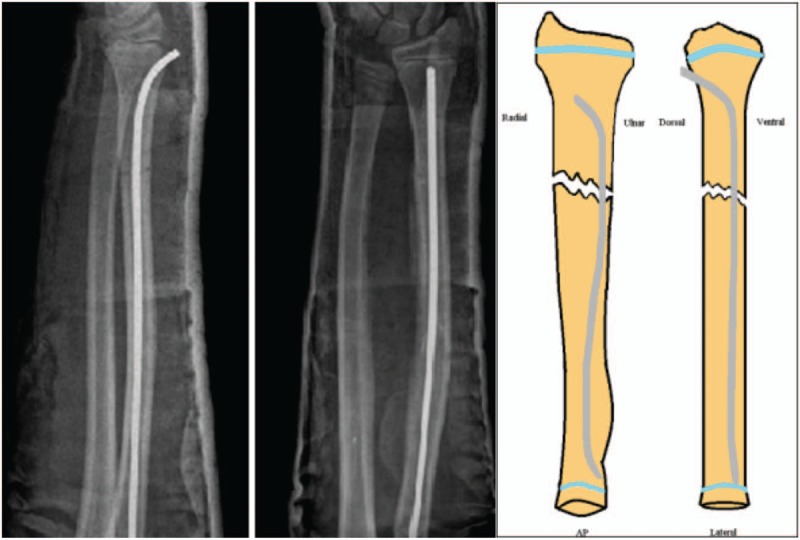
Lateral and anteroposterior radiographs representing the dorsally inserted ESIN (DESIN) technique. DESIN = dorsally inserted titanium elastic stable intramedullary nailing, ESIN = elastic stable intramedullary nailing.
